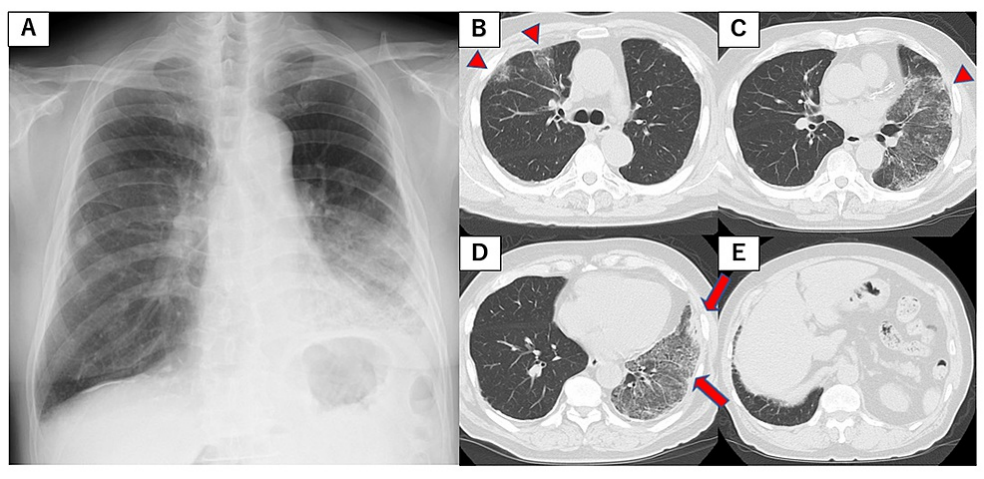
Radiological findings at initial examination. (A) Chest X-ray image. (B–E) Chest CT images showing multiple ground-glass opacities (GGOs) in both lungs (arrowheads), with consolidation and traction bronchiectasis in the left lower lobe (arrows).
